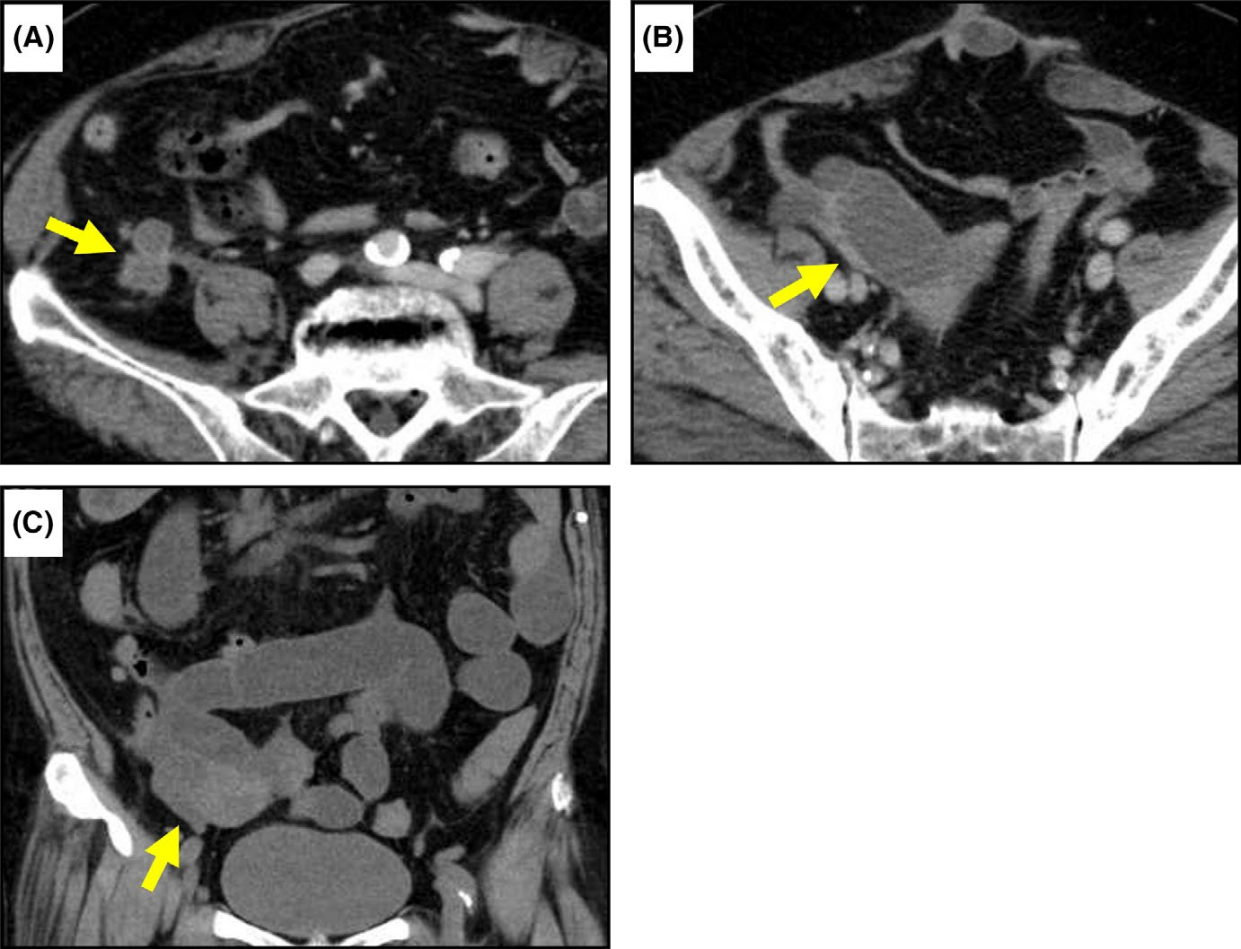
Follow‐up computed tomography (CT) scan showed local recurrence (A) and an enlarged right ovary (B). (C) The patient reported frequent vomiting, and a CT scan showed small bowel obstruction, likely caused by the ovarian tumor, directly infiltrating the small intestine (The arrow indicates the ovarian tumor)
